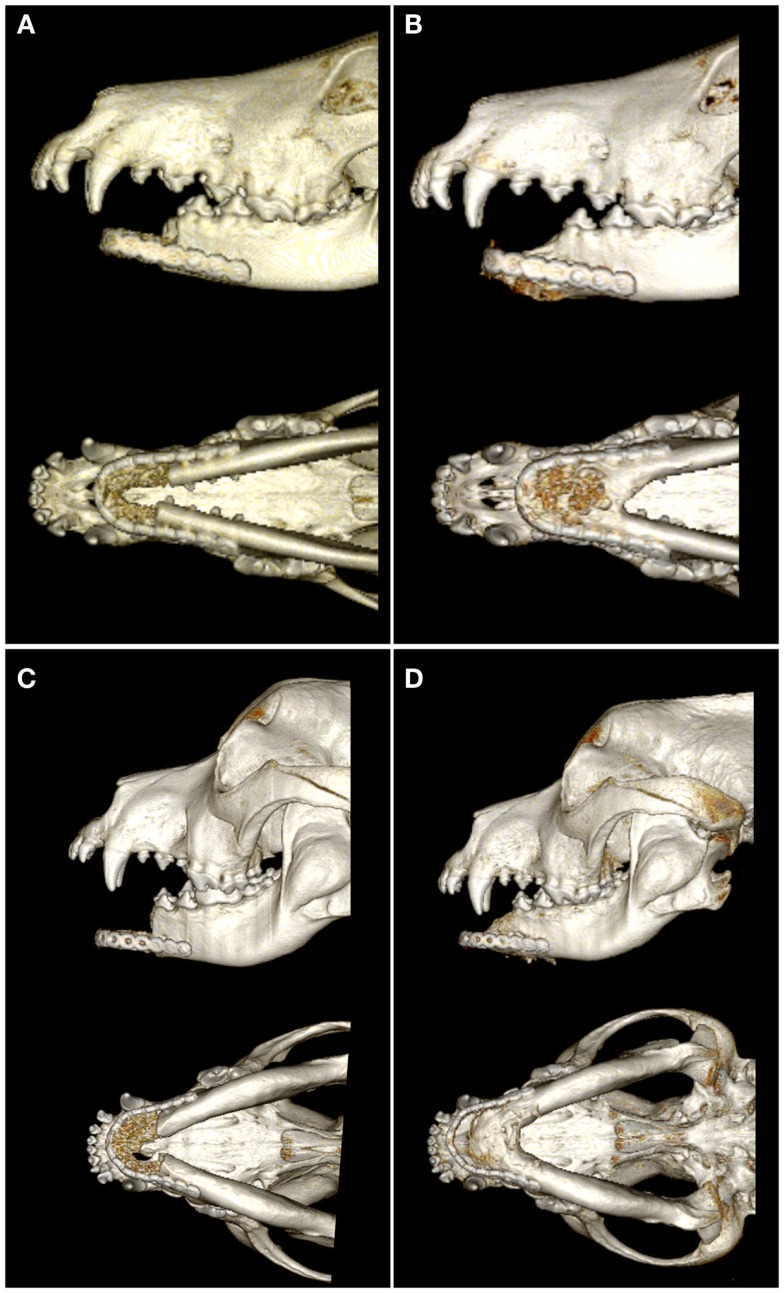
Lateral and ventral views of 3D volume renderings created from the CT images immediately postoperatively (A,C) and 6 months after surgery (B,D). Note the new bone formation in the intermandibular space and bridging the ostectomy sites of both patients 6 months after surgery.
